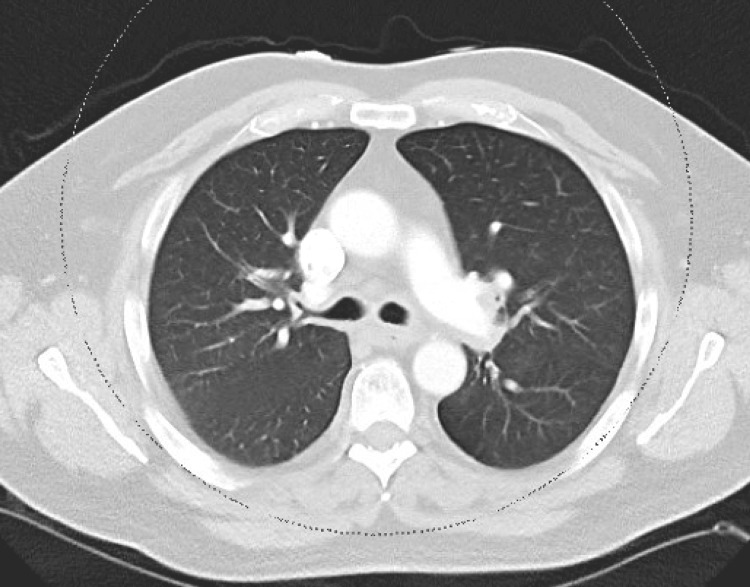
Computed tomography angiography of the chest without evidence of pulmonary embolism.
